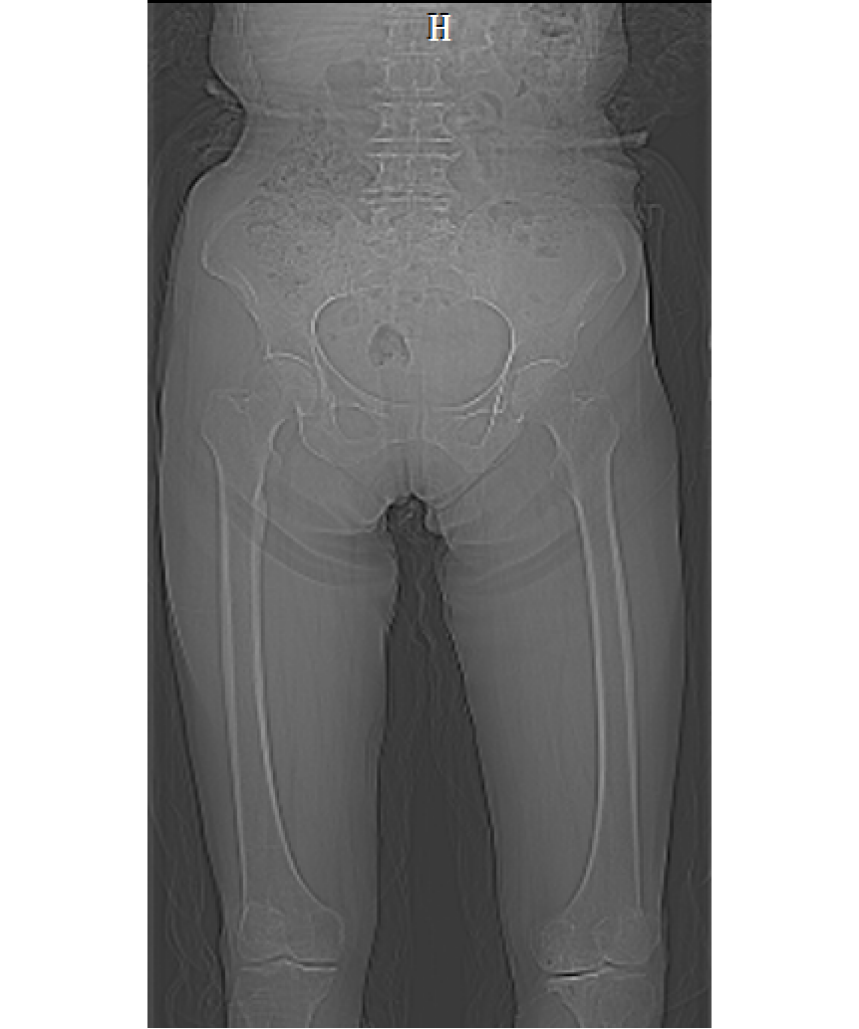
Magnetic resonance image of the older female participant. H: head.
